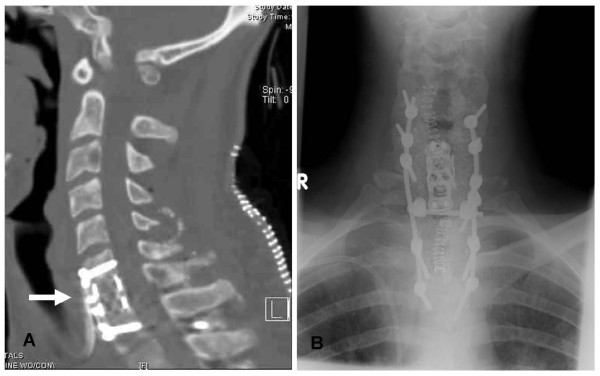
Post-operative CT imaging demonstrates the placement of C7 corpectomy cage (A); Plain X-ray showing ventral anterior plate and corpectomy cage and dorsal hardware, with lateral mass screws, in place (B).
